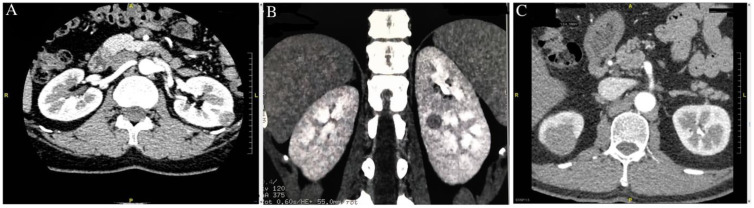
Contrast-enhanced CT of the abdomen. (A) Contrast-enhanced CT scan of the abdomen showing a solid nodule (1.5cm diameter) in the lower pole of the left kidney. (B) Abdominal contrast-enhanced CT showing a solid, circumscribed, low-density cortical lesion (1.3cm diameter) in the posterior lip of the left kidney. (C) Contrast-enhanced CT scan of the abdomen showing a soft tissue mass in the upper pole of the right kidney (3.2cm diameter).
